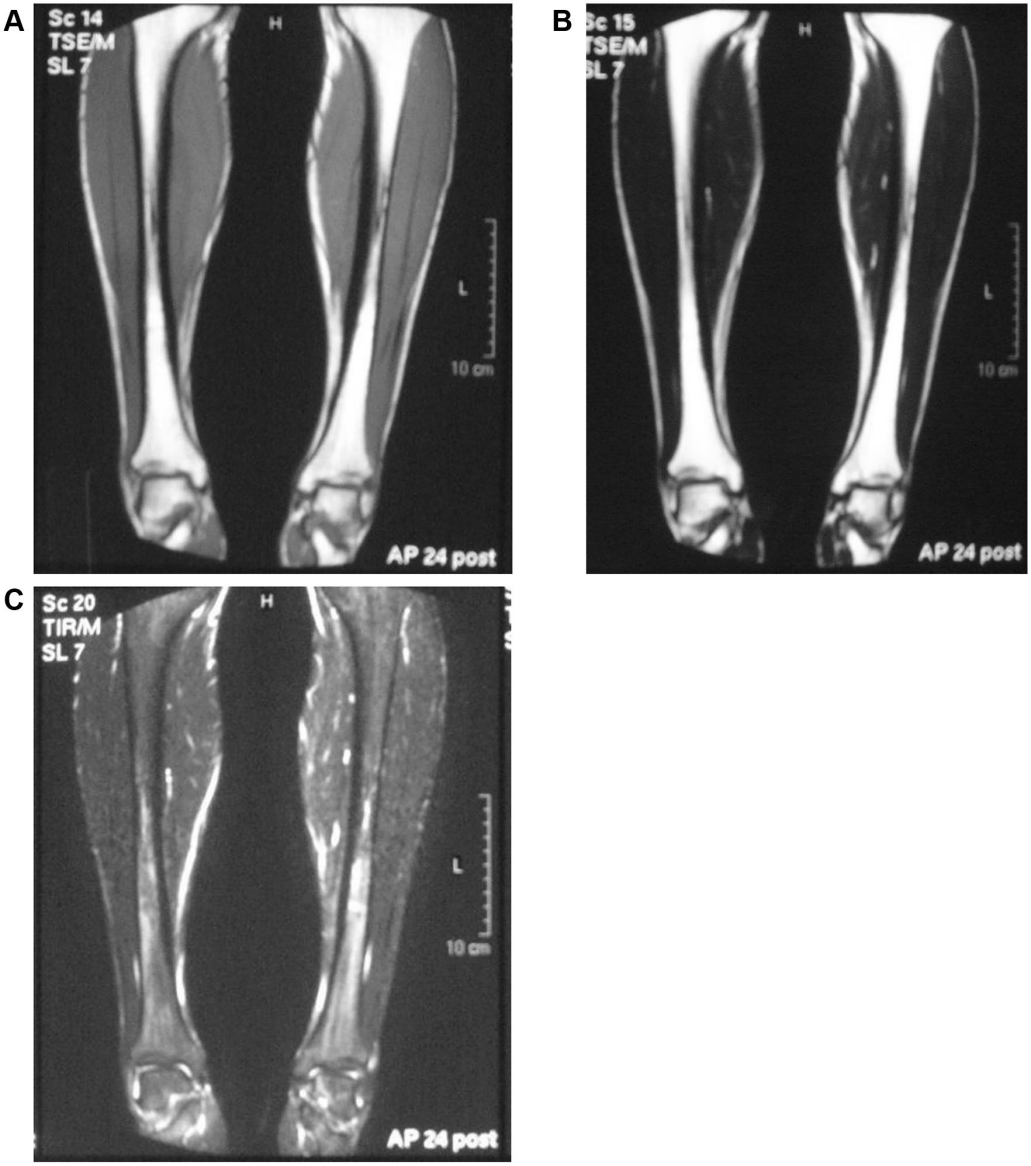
Initial coronal MRI scans diagnosed with a stress fracture show a strikingly wide low-signal intensity on the T1-weighted scan (A), and a high-signal intensity on the T2-weighted scan (B) and STIR fat-suppressed scan (C) in the localized bone marrow. The abnormal finding is more detectable on the STIR fat-suppressed MRI scan
